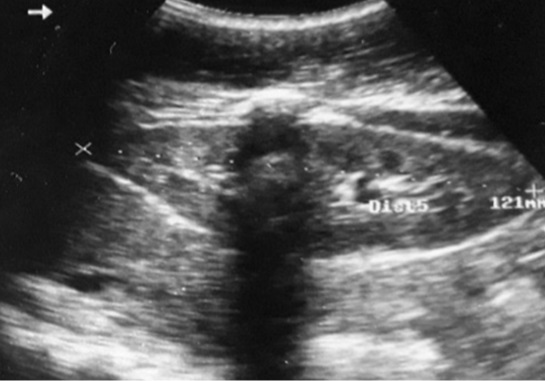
Ultrasound image of enlarged kidney. Right kidney measuring 121 mm in length with good corticospinal differentiation.
